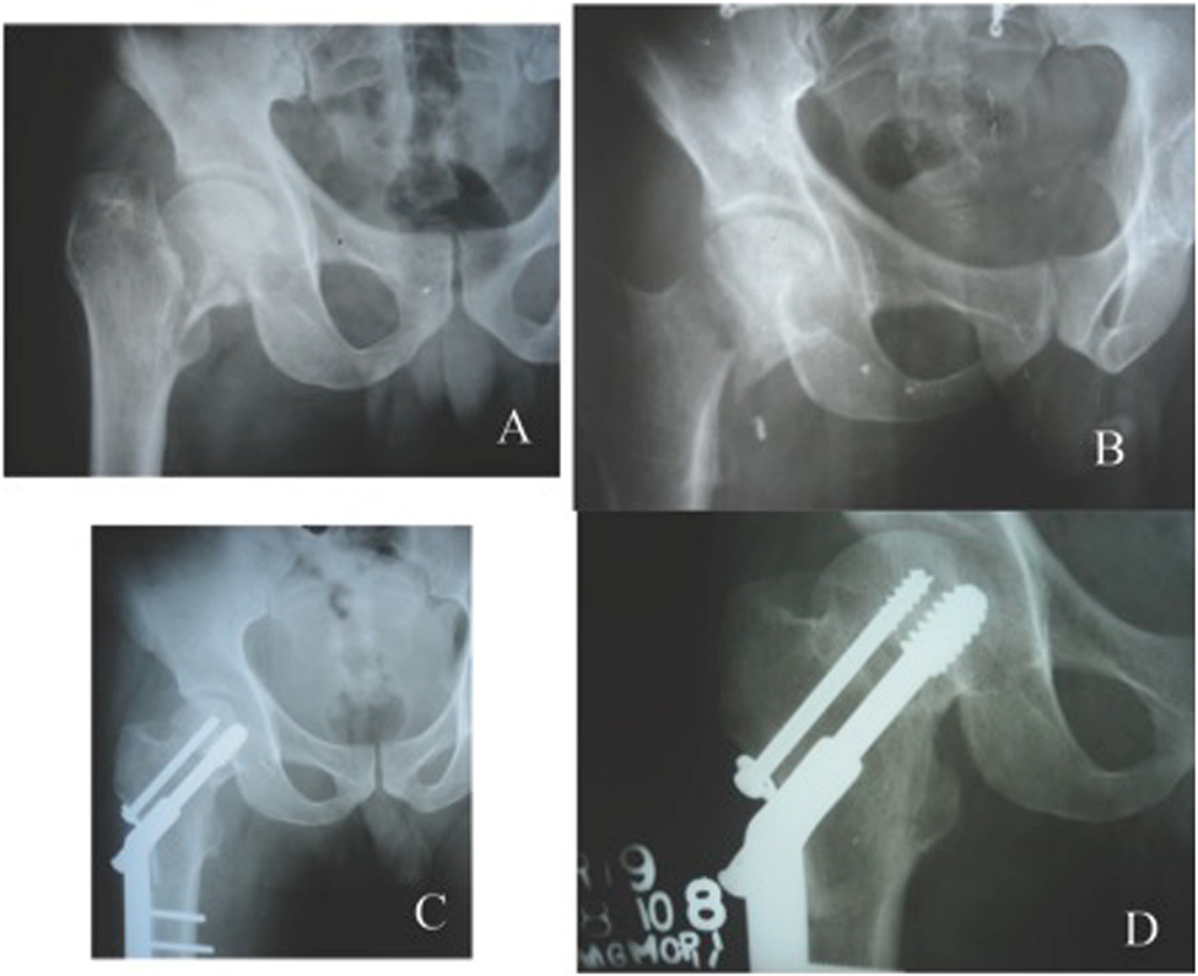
Varus nonunion of an intracapsular neck fracture in a 37 year old male. Post operative radiographs showing good union at the nonunion site and at the osteotomy site with restoration of the neck shaft angle.
